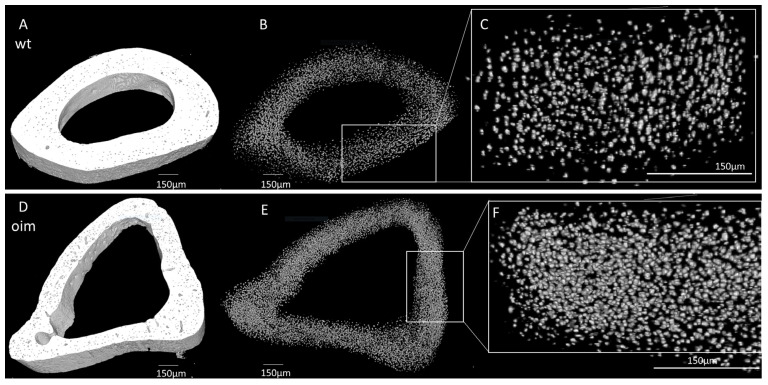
Nano-CT analysis of humerus diaphysis of WT and OIM. (A,D) Binarized reconstruction of humerus midshaft. (B,E) 3D reconstruction of the discriminated binarized osteocyte lacunae throughout the midshaft segment. (C,F) Magnification of 3D reconstruction of the osteocyte lacunae.
